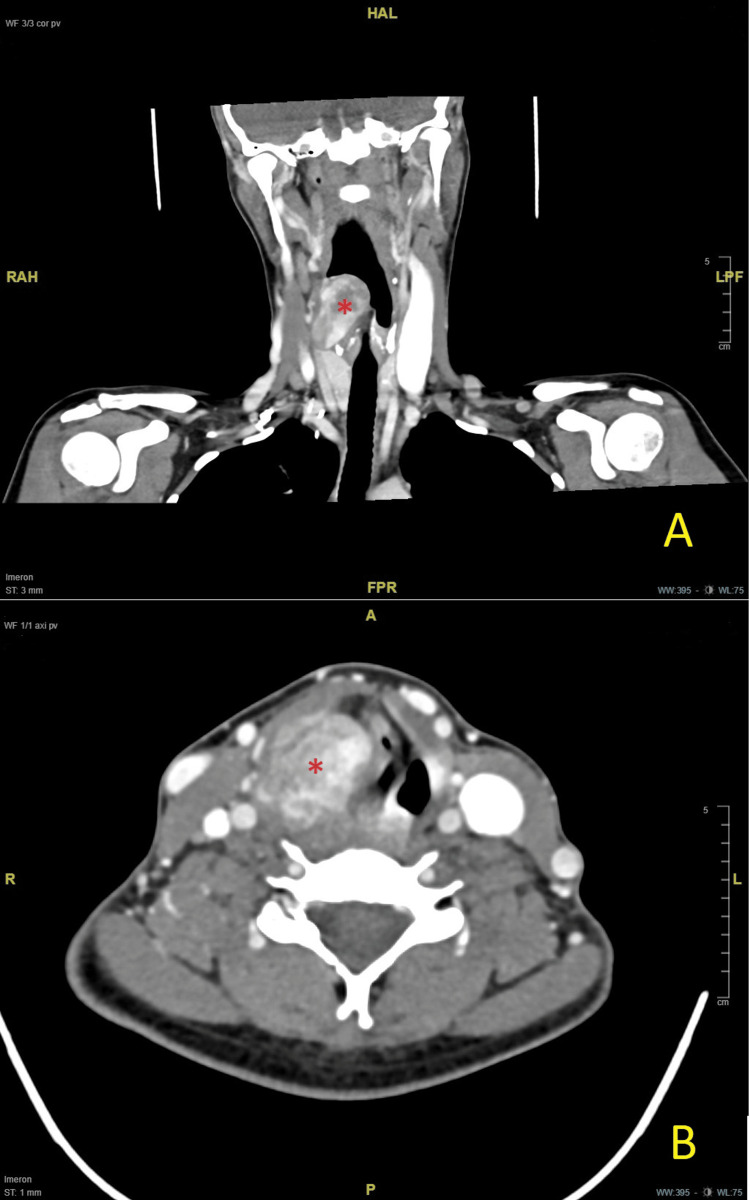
Preoperative CT scans. (A) Coronal view; (B) axial view. *Solitary fibrous tumor attached to the right pyriform sinus, obstructing the larynx.
